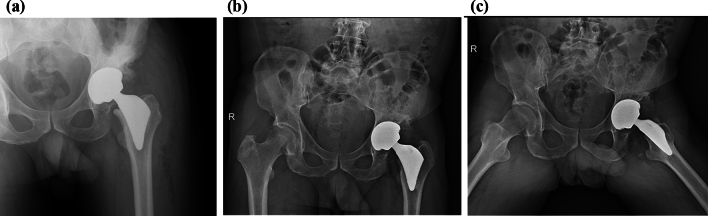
a Anteroposterior radiograph of the same patient on the first day after cementless total hip arthroplasty with the Proxima stem, placed in a neutral position (alignment). b Anteroposterior radiograph of the same patient, 12 years postoperatively, osseously stable stem fixation with bony trabecular development in modified Gruen zones 2 and 6 (around the distal part of the stem) and stress-shielding phenomenon in modified Gruen zone 7. cAxial radiograph of the same patient 12 years after surgery with visible bony trabecular development around the distal part of the stem
